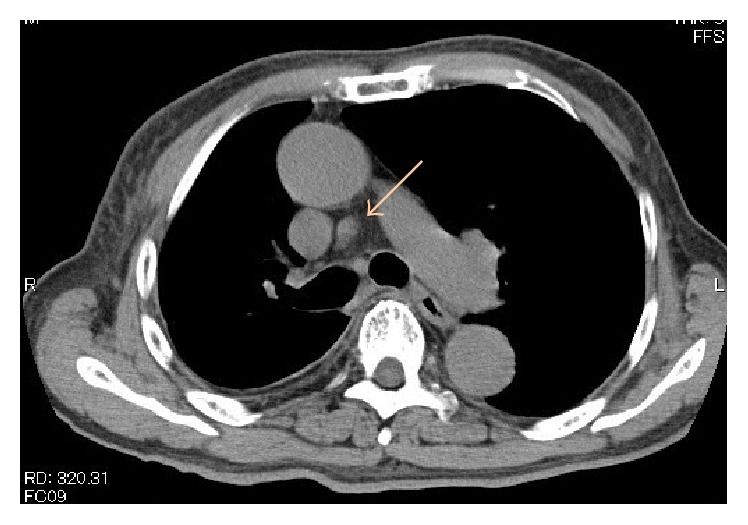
Mediastinum computed tomography.
